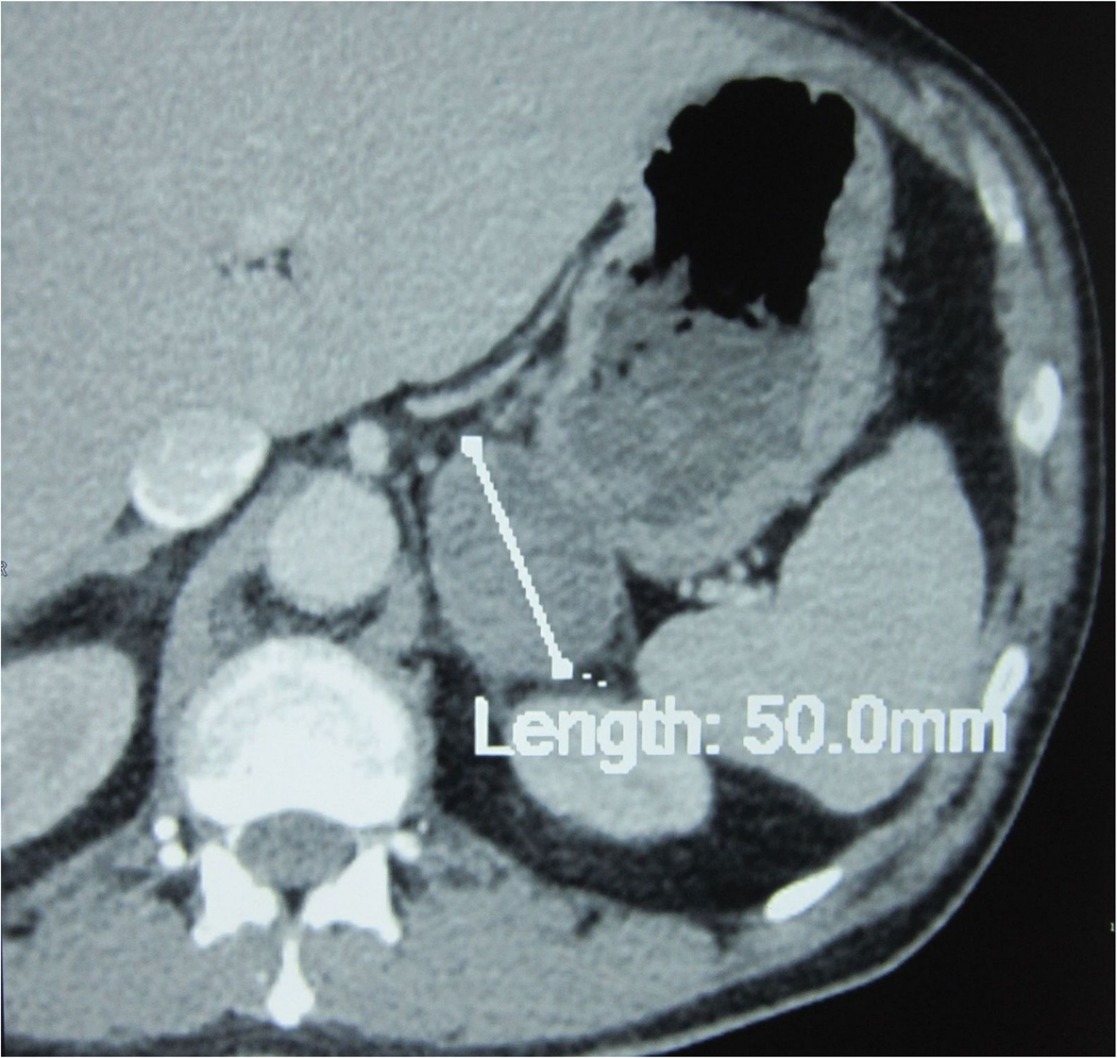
Control abdominal CT showing left adrenal gland metastasis
